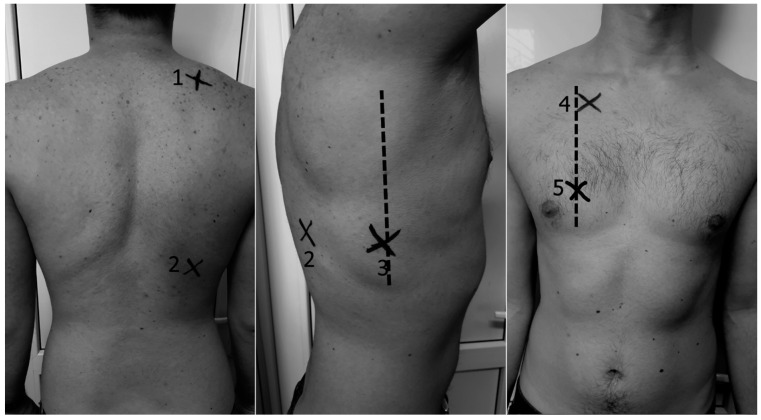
Lung intercostal spaces assessment in a reduced lung ultrasound (LUS) protocol (10 lung intercostal spaces). (1) Posterior suprascapular intercostal space (IC); (2) posterior basal subscapular IC (space 5–7); (3) lateral basal 5–7 IC along midaxillary line; (4) anterior 2 IC along midclavicular line; (5) anterior basal 5–7 IC along midclavicular line.
